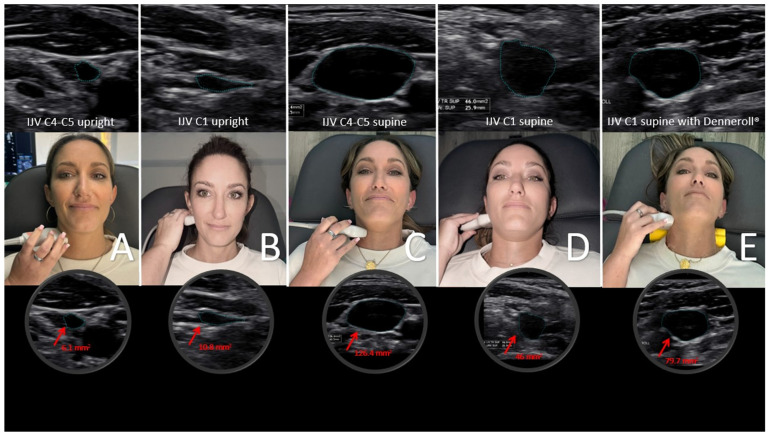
Internal jugular vein (IJV) cross-sectional area (CSA) measurement using ultrasound in various head and neck positions. (A) Upright measurement at the C4–C5 level. (B) Upright measurement at the atlas (C1) level. (C) Supine measurements at the C4–C5 level while lying on the Denneroll^®^. (D) Supine measurements at the C1 level. (E) Supine measurements at the C1 level while lying on the Denneroll^®^. The internal jugular vein is typically much more open in the supine position compared to upright, and when there is a breakdown of the cervical curve (dysstructure) and/or ligamentous cervical instability, the IJV gets maximally compressed at the atlas. Figure copyright belongs to authors. (Reproduced from Ref. [29]).
